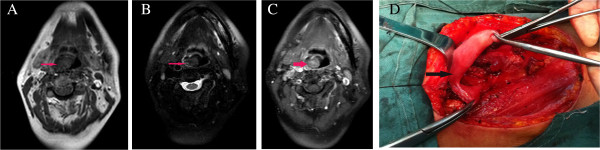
Magnetic resonance imaging revealed a 1.3 × 2.2-cm mass in the right pyriform sinus. (A) Signals were isointense and slightly hypointense on the T1-weighted imaging, (B) hyperintense on T2-weighted imaging, and (C) heterogeneous enhancement was observed on the contrast-enhanced T1-weighted MRI images. (D) The patient underwent complete resection via a lateral neck incision and achieved a clear margin at second surgery.
